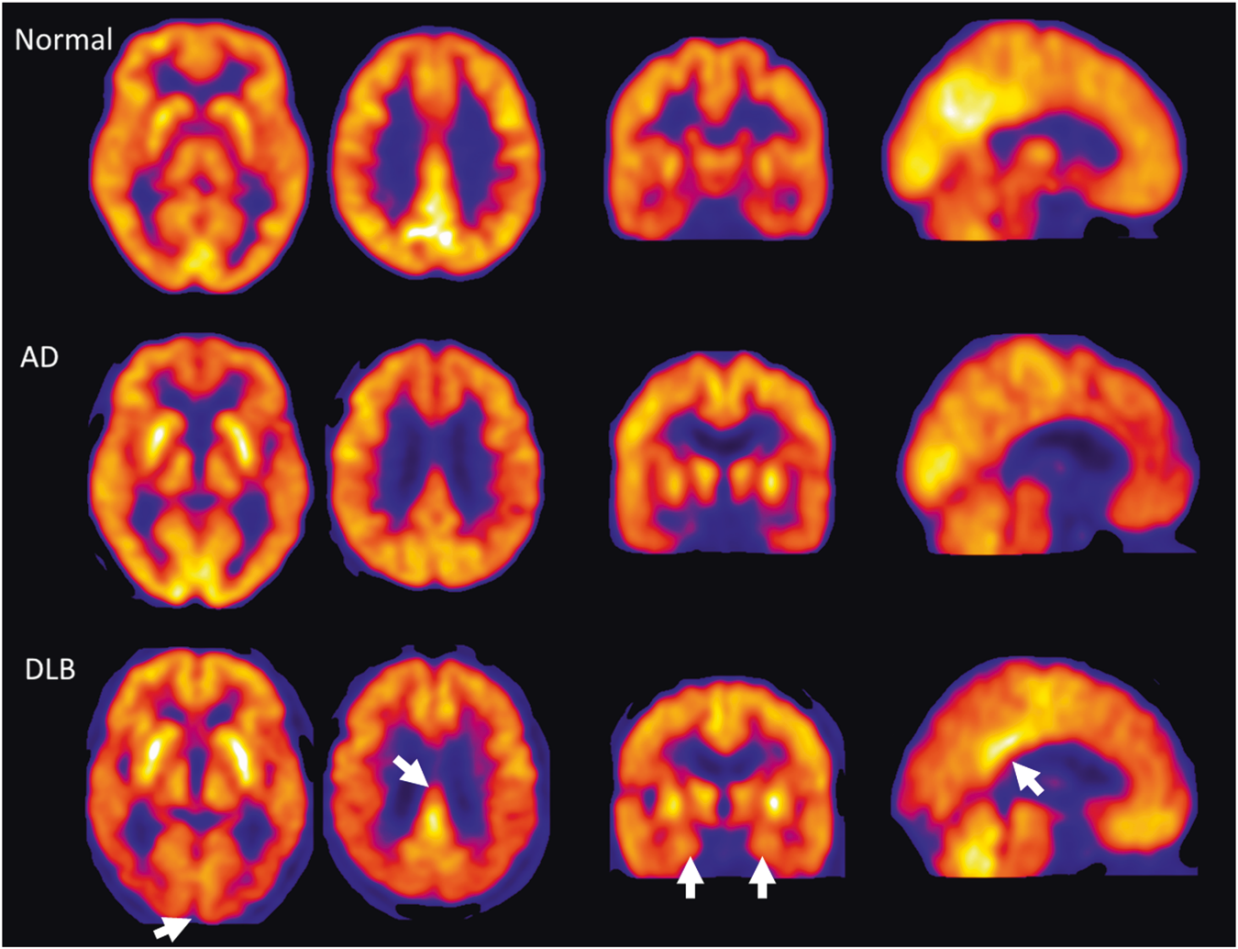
^18^F-Fluorodeoxyglucose (FDG) PET in Alzheimer’s disease (AD) and Dementia with Lewy Bodies (DLB). FDG PET representative images showing reduced local cerebral metabolic rate of glucose consumption in cases of AD and DLB compared to a non-demented control study participant. The white arrows highlight the relative preservation of the hippocampus and posterior cingulate gyrus in DLB compared to AD and the occipital hypometabolism in DLB. These are FDG PET scans from the Study of the clinical utility, patient preference and cost benefit of SPECT and PET-CT brain imaging in the evaluation and diagnosis of Alzheimer’s Disease (Suspected-AD). Images are courtesy of Dr Michael Firbank, Newcastle University, UK.
